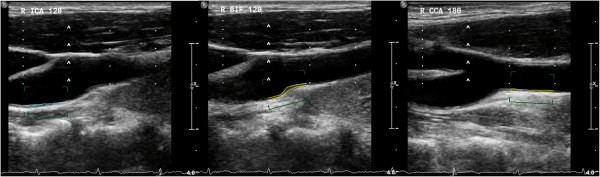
Ultrasonographic IMT measurement in the proximal ICA (left), the bifurcation (middle) and the distal CCA segment (right) by QLAB software.
